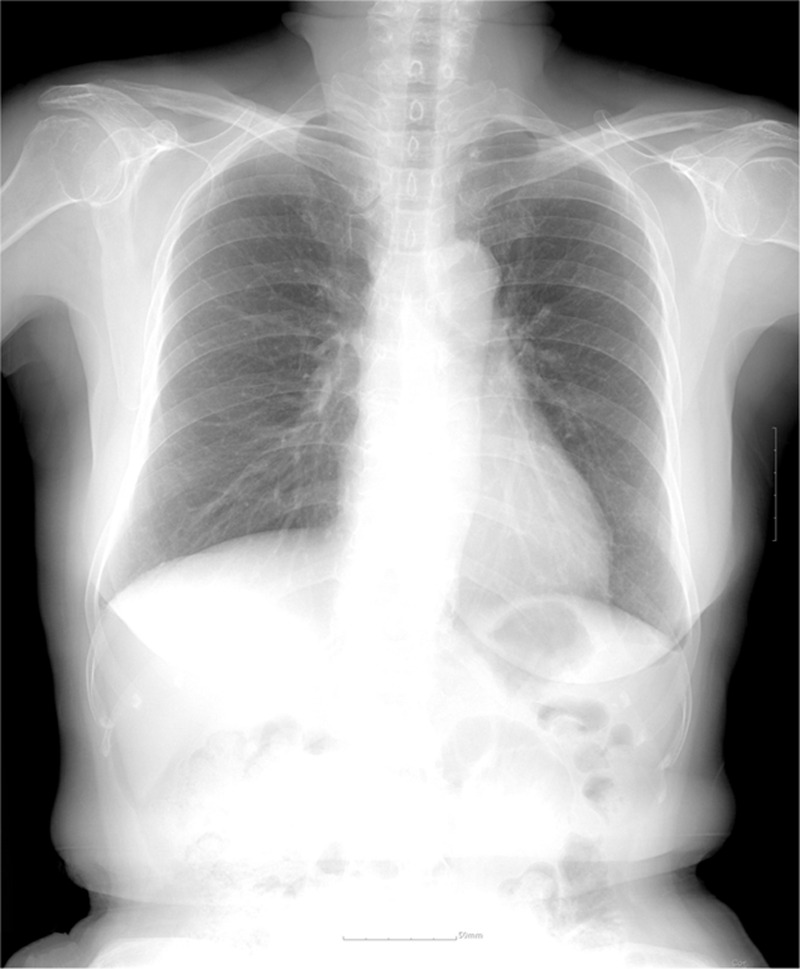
Chest X-ray at 15 months postoperatively showing fully expanded lungs.
